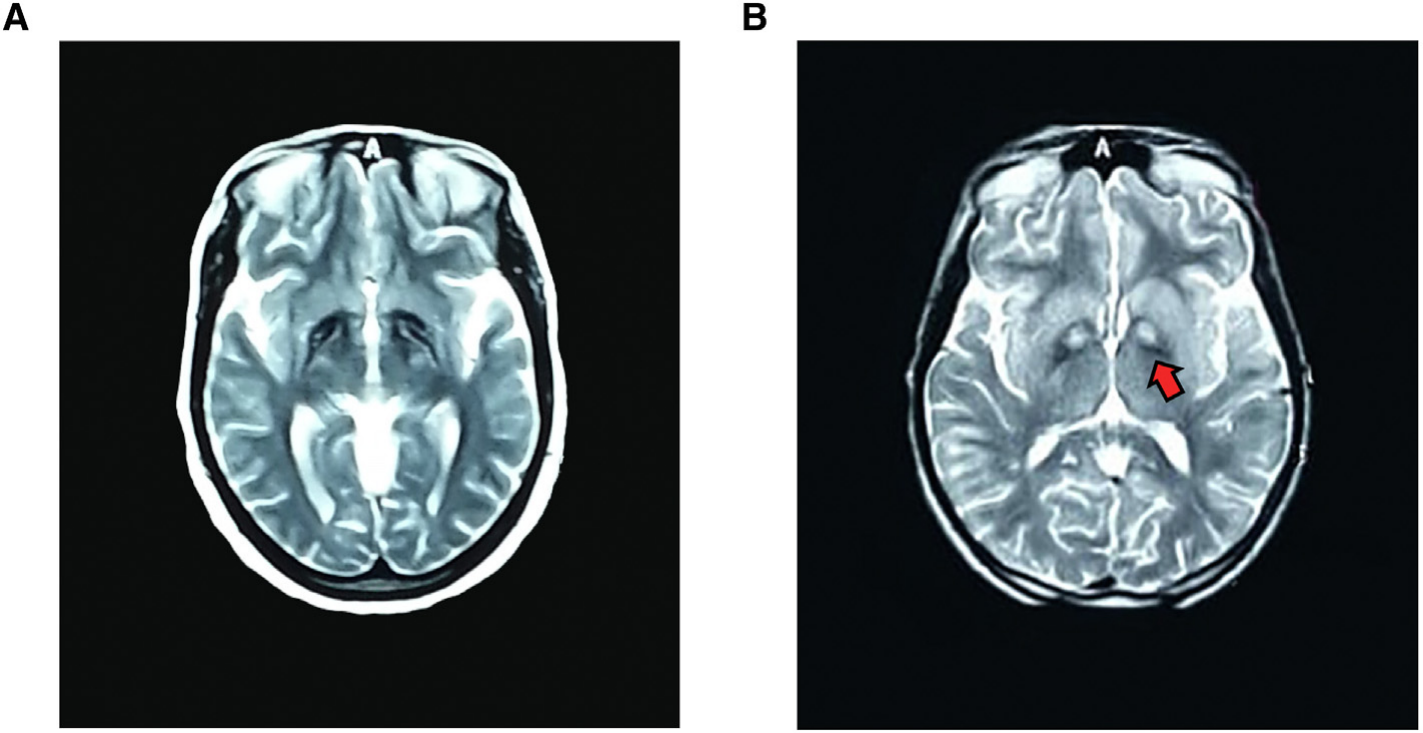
Brain MRI scans of PKAN-affected family members. Images show axial brain MRI scans of patient II.3 (A, FLAIR) and II.10 (B, T2WI) with hyperintensity surrounded by hypointensity of globus pallidus with the characteristic eye-of-the-tiger sign (red arrow).
